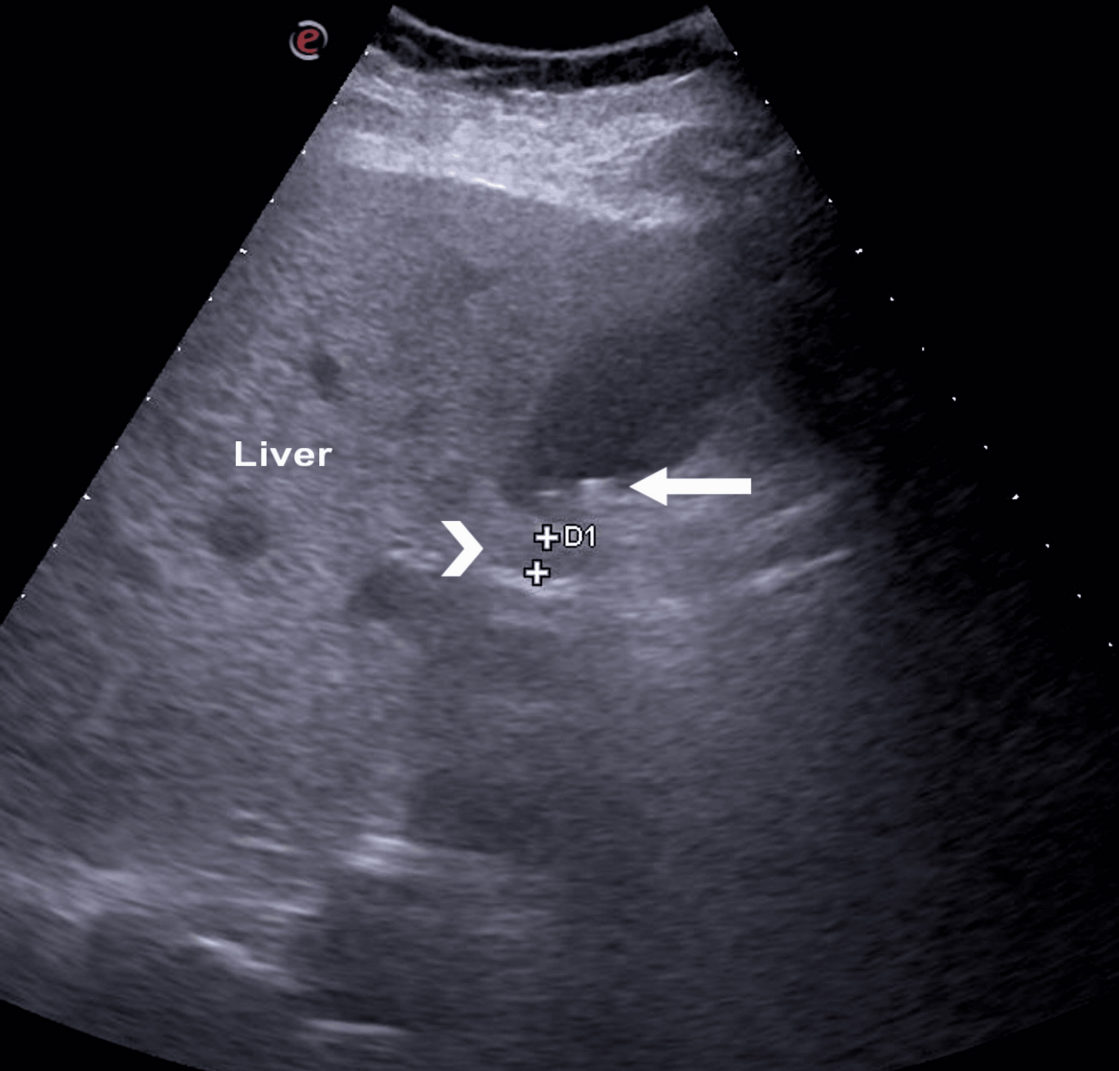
Ultrasound image showing the liver, multiple sub-centimeter gallstones (solid arrow), and the common bile duct (arrowhead).
